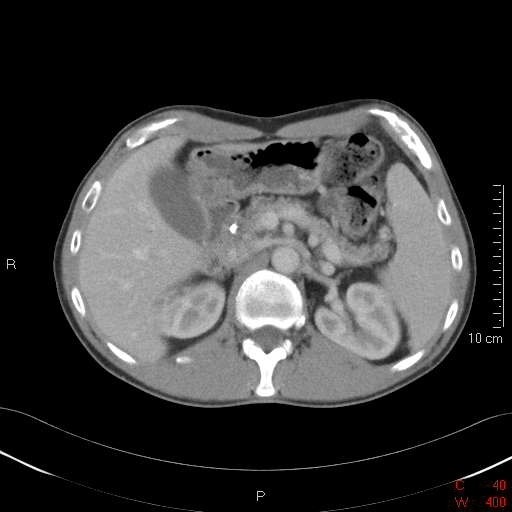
Computed tomography scan at 18 months showing resolution of the pancreatic inflammatory mass.
